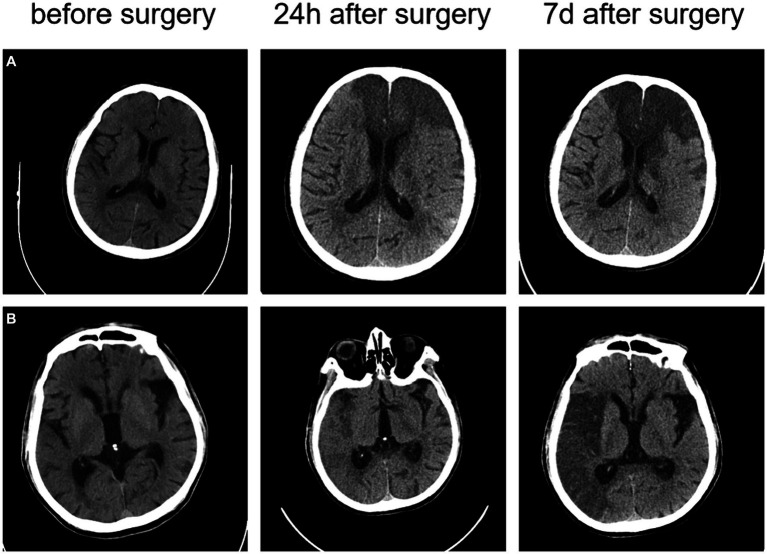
The CT images of two ischemic stroke patients undergoing thrombectomy (A) a 73-year-old male patient with an NIHSS score of 24 at the onset; and (B) a 78-year-old male patient with an NIHSS score of 14 at the onset.
